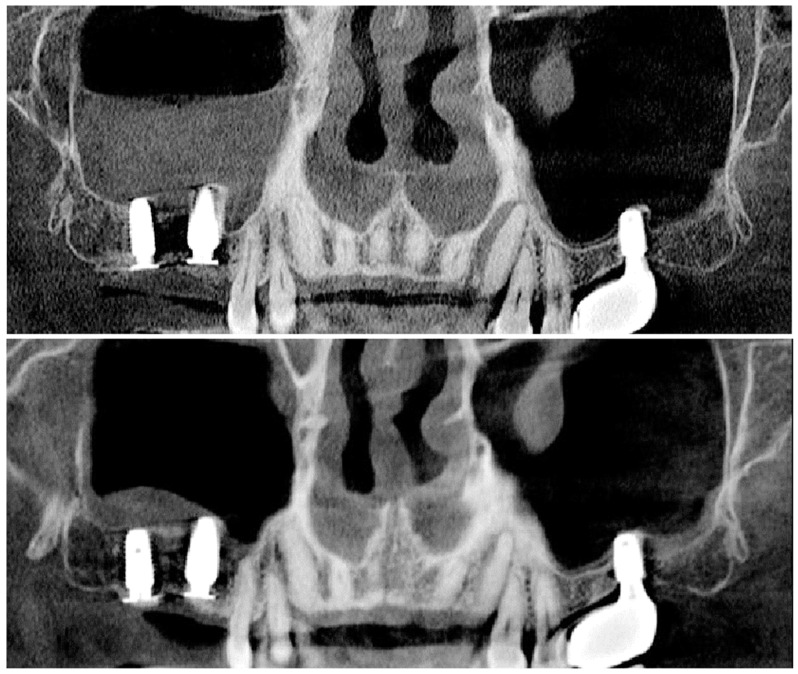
(a) Preoperative panoramic image of CBCT scan indicates mucosal elevation and bone grafting in the defect. (b) Panoramic image of CBCT scans after 5 months of healing reveals the resolution of a huge cyst and autologous bone reformation in the sinus. Successful ridge augmentation was also revealed.
